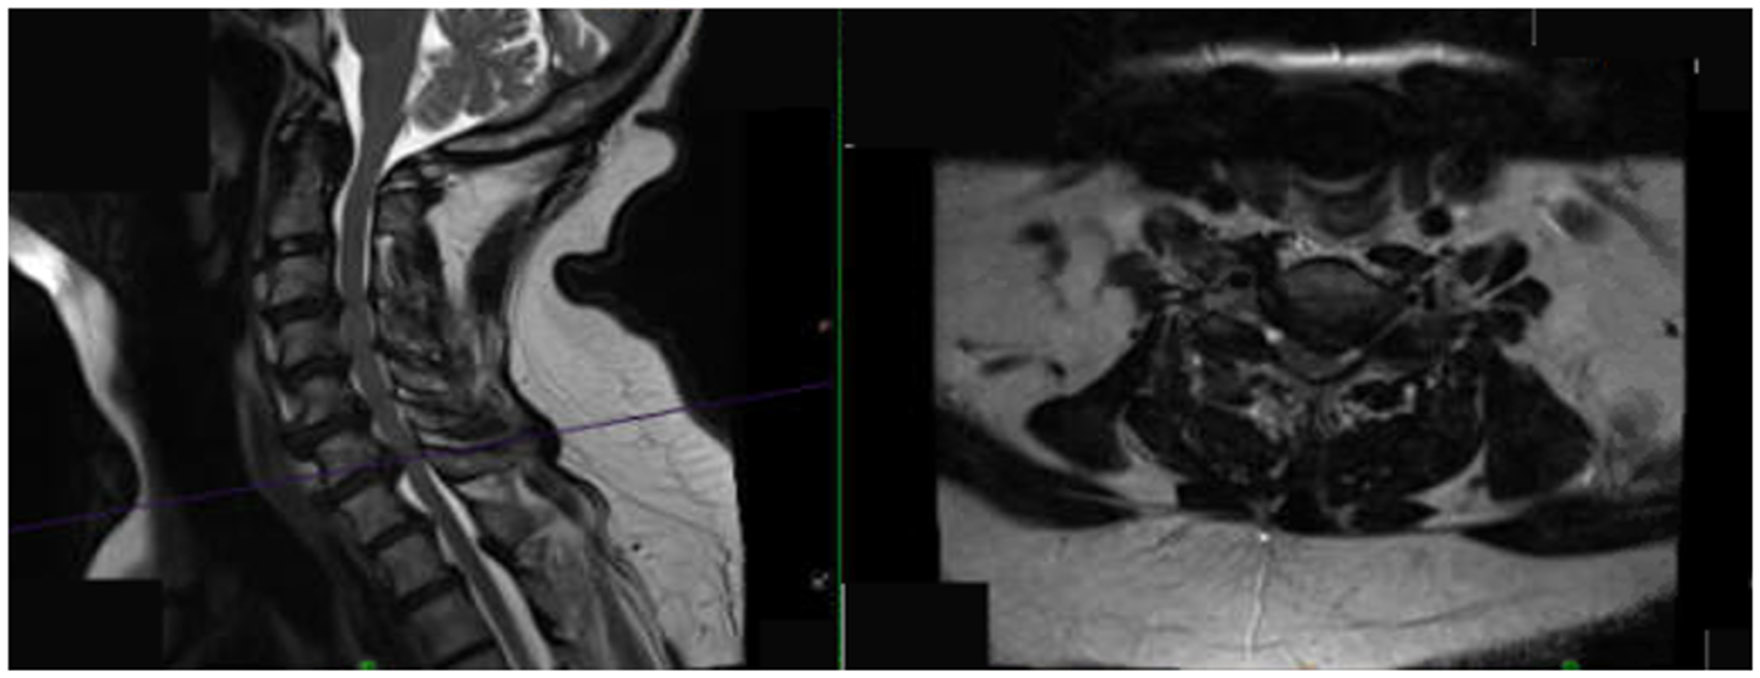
MRI of cervical spine demonstrating levels of degenerative disc disease and levels of vertebral canal stenosis secondary to ossification of the posterior longitudinal ligament at the vertebral canal, most significant at C6-7.
